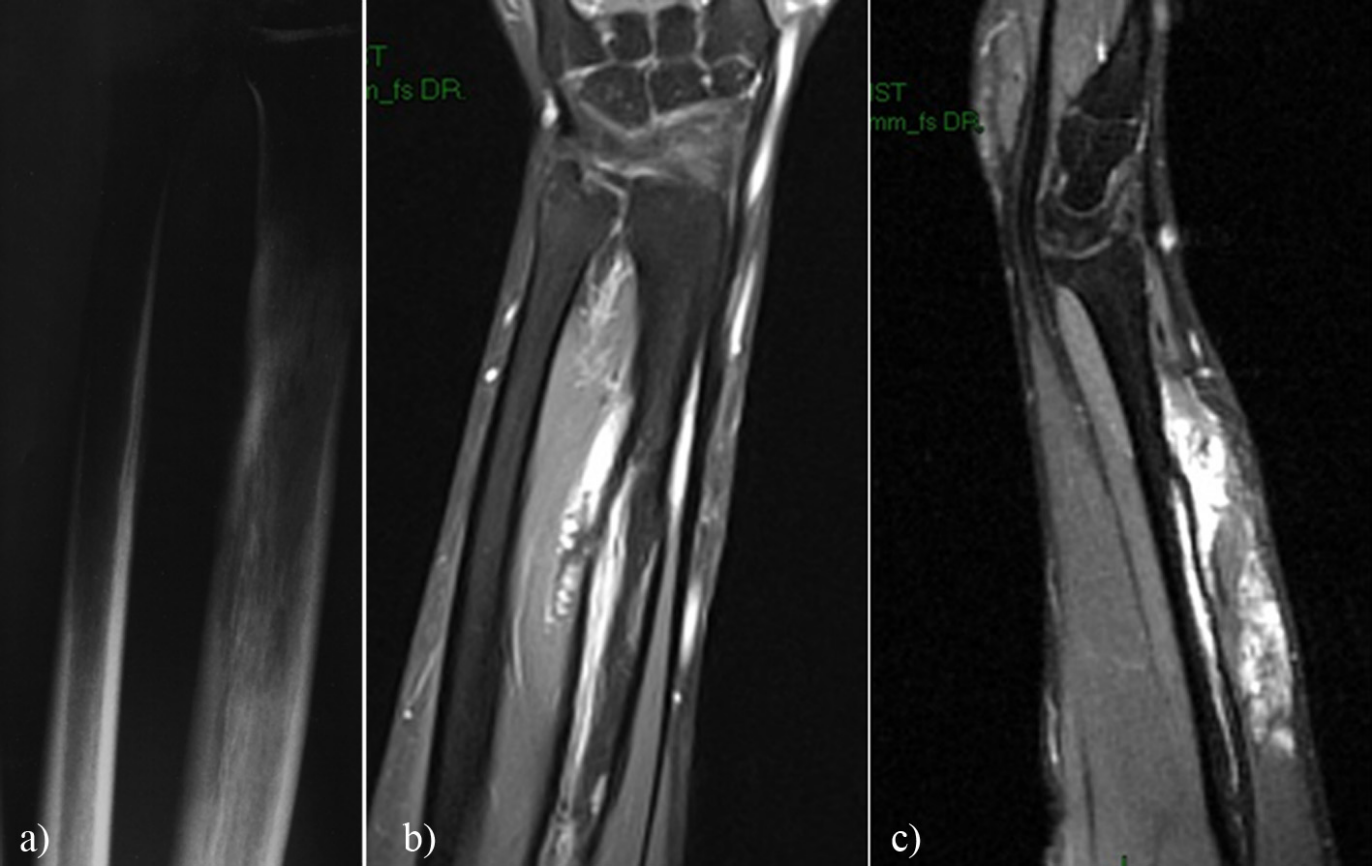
X-ray and MRI forearm examination. 1.a. AP radiograph of the distal forearm. Lamellar periostosis of the radius bone associated with diffuse bone structure change and an area of reduced bone density (a) are seen. 1.b, c. MRI forearm examination. Sagital FS T1 shows inhomogeneous tumor with irregular contours with diffuse post contrast enhancement and osseous invasion (b). MRI coronal section T1TSEfs shows post contrast better highlighting of the osseous invasion (c).
